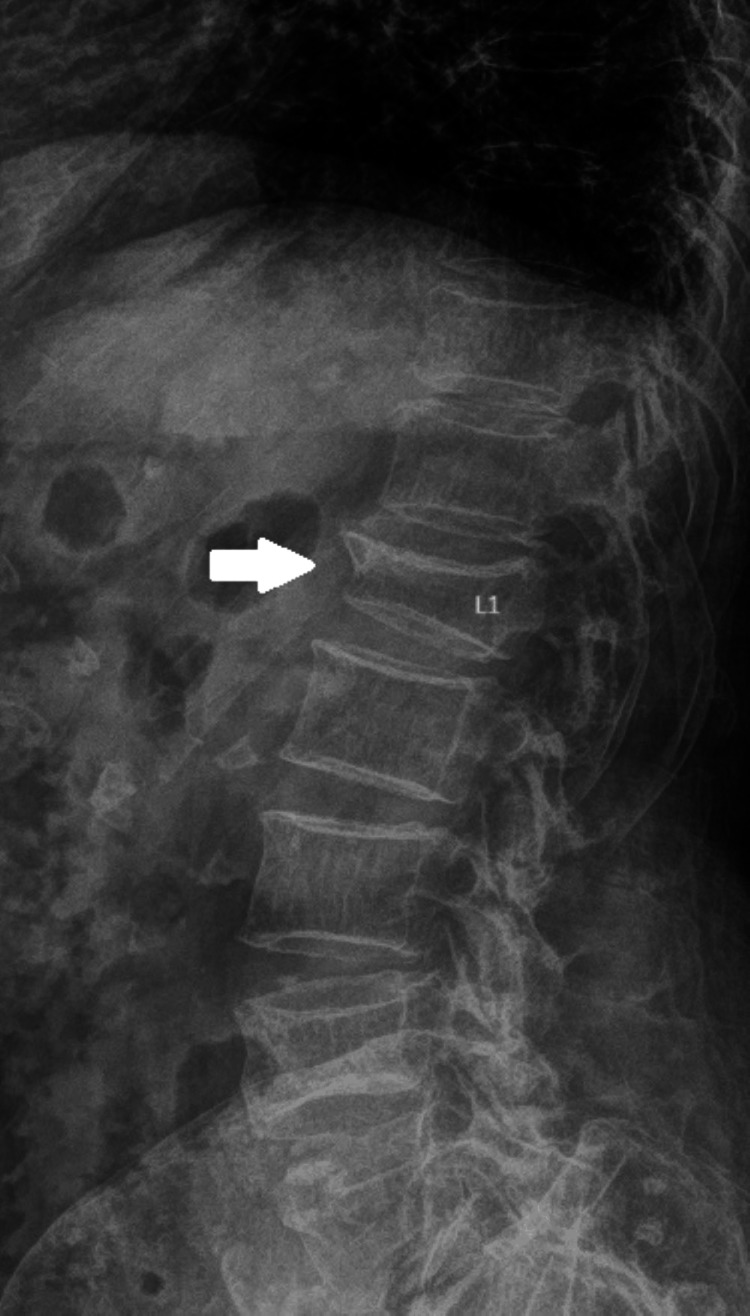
Preoperative radiograph (lateral view) of the lumbar spine. The white arrow depicts the osteoporotic vertebral fracture (OVF) of L1.
